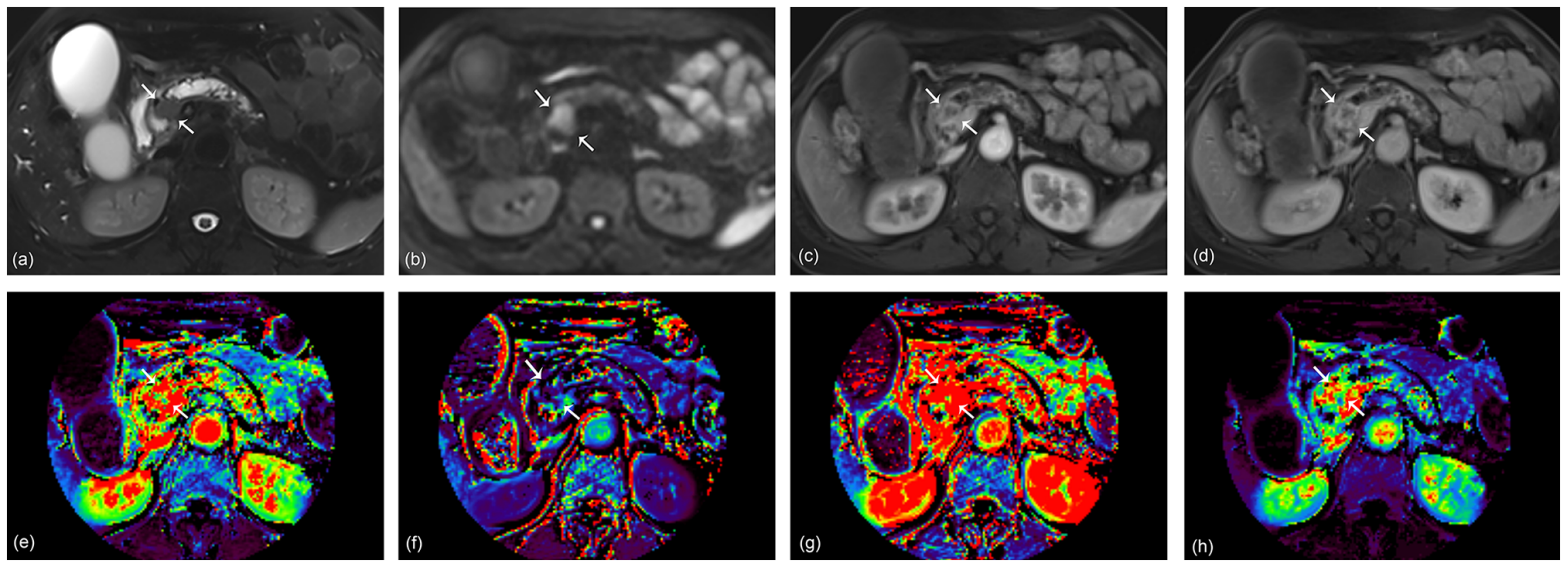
A 68-year-old woman with pancreatic ductal adenocarcinoma in the pancreas head. A 2.3 cm tumor shows high signal intensity on axial T2-weighted image (A) and diffusion-weighted image (B). Arterial (C) and portal (D) phase images of dynamic contrast-enhanced (DCE) MRI show rim enhancement of the tumor. K^trans^
